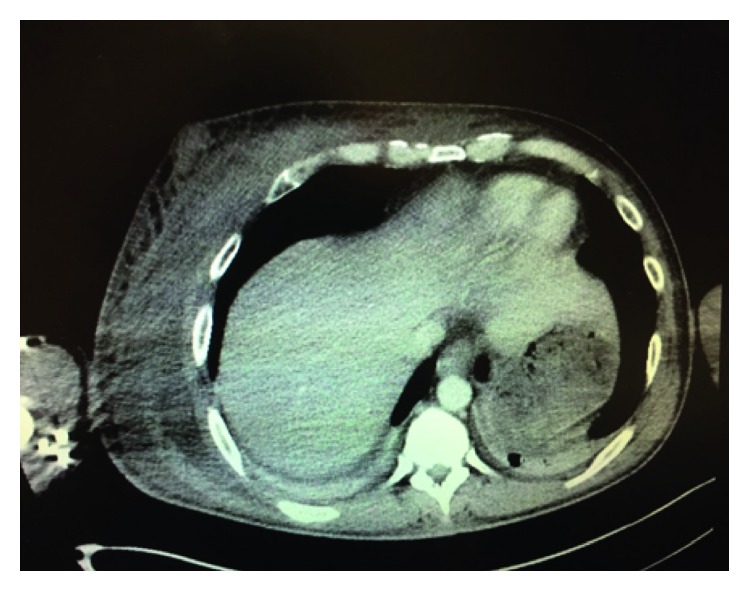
CT scan of chest with contrast at the level of the pectoralis muscle.
